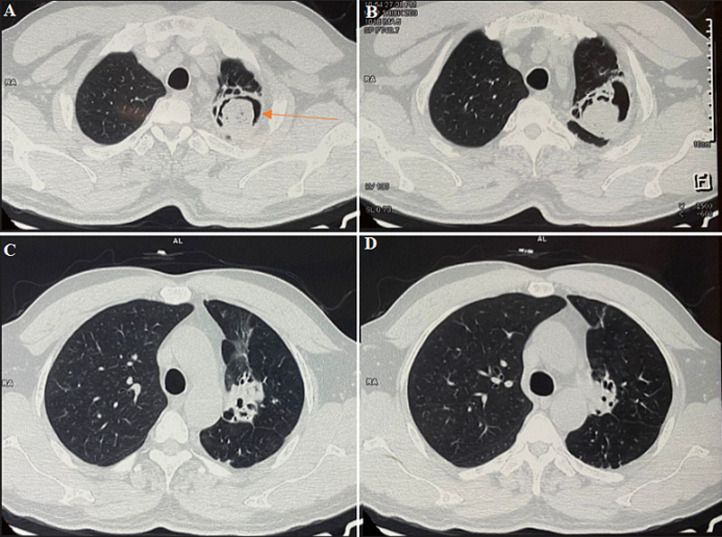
A,B,C,D) high resolution computed tomography (HRCT) of the thorax showing air crescent sign
